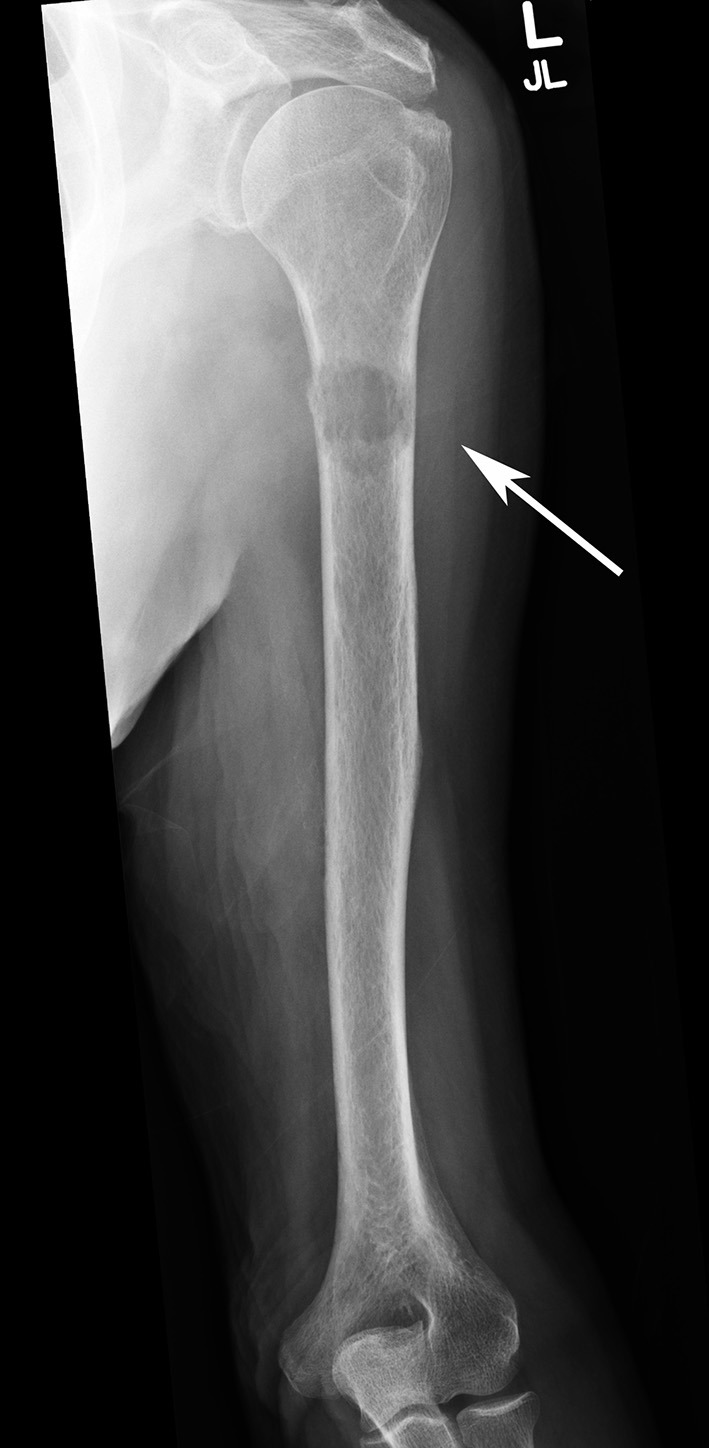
AP radiograph of a left humerus demonstrating a lytic metastatic lesion (arrow) in the proximal diaphysis. Note the extensive cortical involvement, predisposing it to a pathological fracture.
